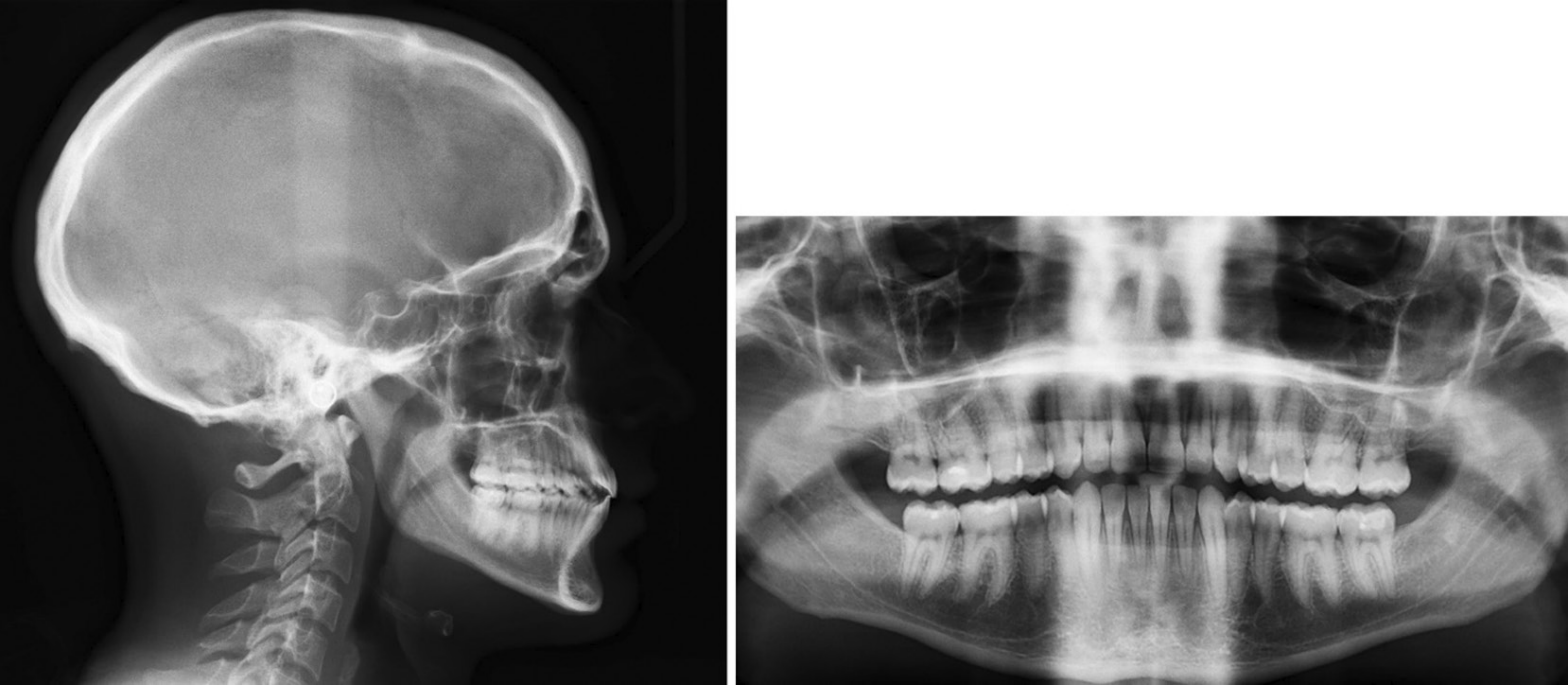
Post‐treatment radiographs
